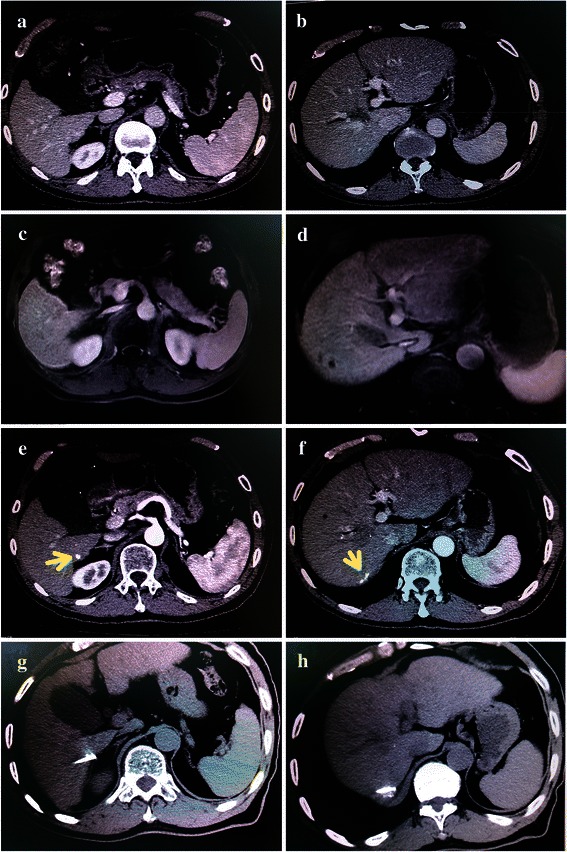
Perioperative imaging of patients with HCC. a and b, viable lesions are not shown in different segments of the liver via preoperative CT. c and d, it is very difficult to locate viable lesions in segments 6 and 7 of the liver via preoperative magnetic resonance imaging. e and f, two additional tiny lesions with lipiodol depositions were detected in segments 6 and 7 of the liver (*arrows*) by using lipiodol CT after TACE. g and h, two cancerous lesions were treated with radiofrequency ablation
